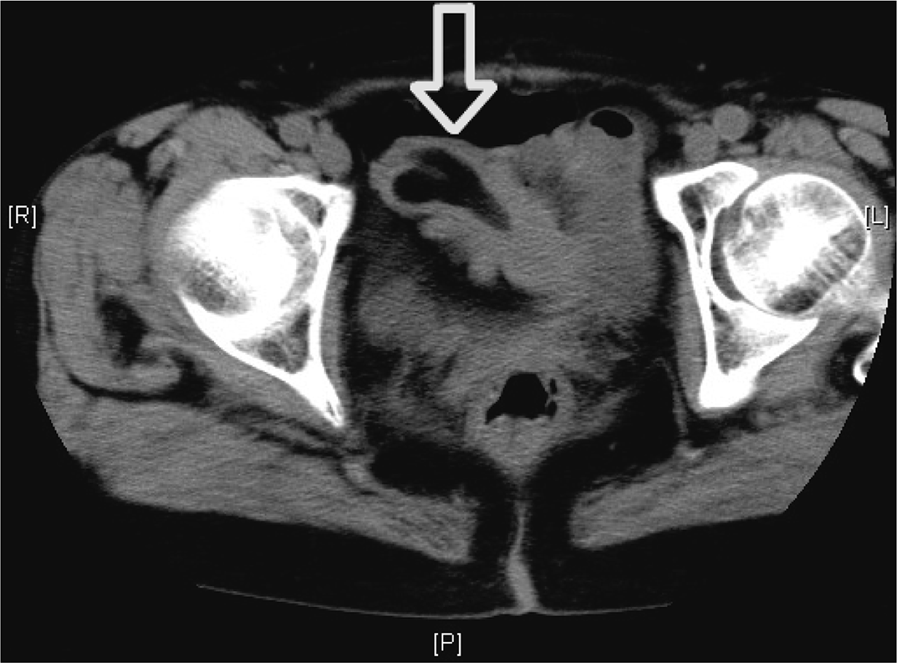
Abdominal computed tomography. Abdominal computed tomography showed a 3.0-cm low-density tumor in the ileum, suggesting a diagnosis of intestinal lipoma.
